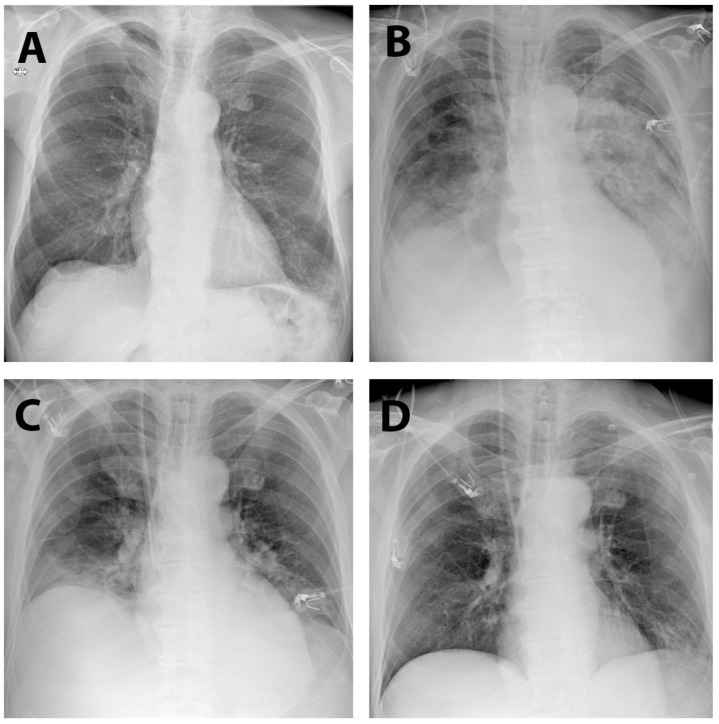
Chest X-ray series: preoperative aspect (A), during acute respiratory failure (B), improvement in lung infiltrates (C), and at discharge (D).
